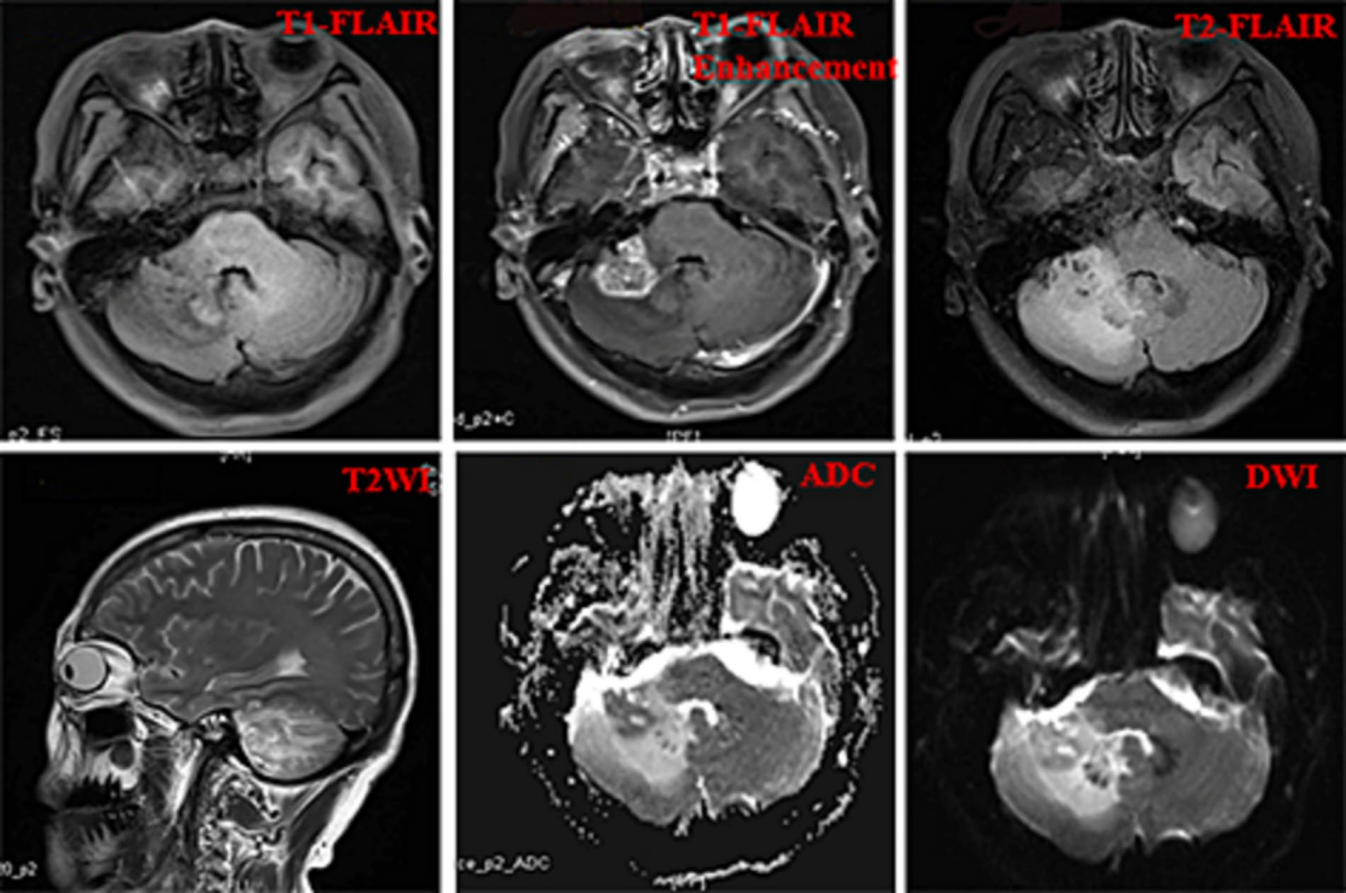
The imaging results of our hospital including T1Flair, T1Flair enhancement, T2Flair, T2WI, ADC, and DWI images.
